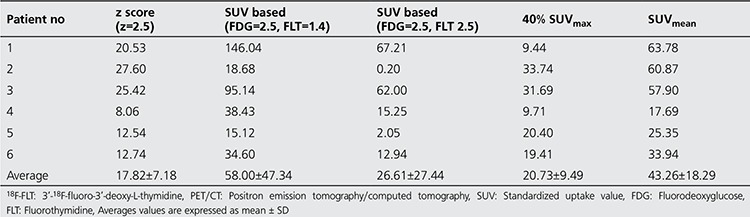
Estimated pancreas tumor volumes using (B) 18F-FLT PET/CT Imaging
